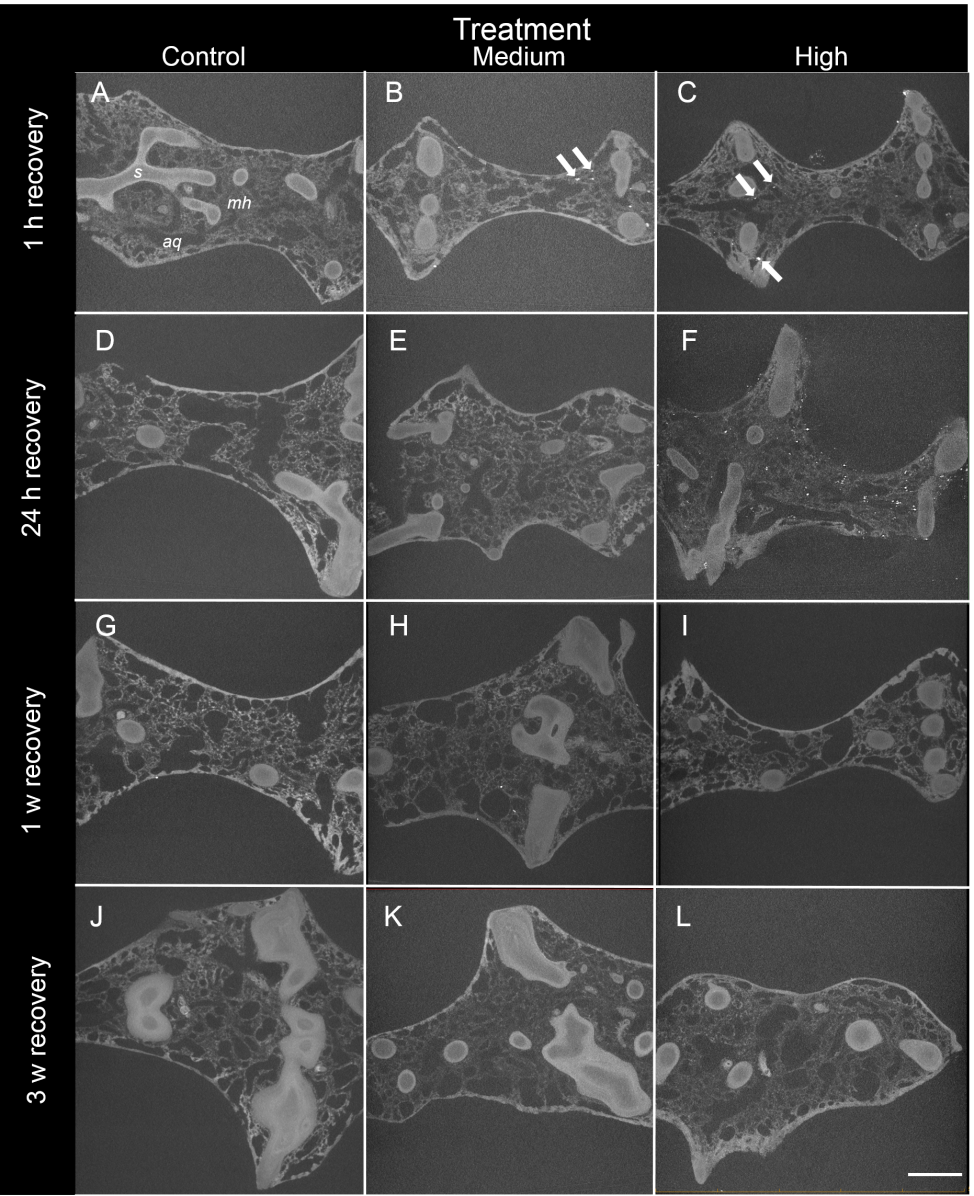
Representative 2D X-ray cross-sections of *I. basta* during the recovery period after 1 h, 24 h, 1 w, and 3 w for the control (0 mg L^−1^), medium (42 ± 8 mg L^−1^), and high siliciclastic sediment (135 ± 30 mg L^−1^) treatments. The sponge skeletal fibres (*s*), mesohyl (*mh*) and aquiferous system channels (*aq*) are visible in all treatments. As recovery time increases, the sediment (white particles indicated by arrows) becomes less concentrated, and no sediment was observed in the control sponges (scale = 1 mm).
